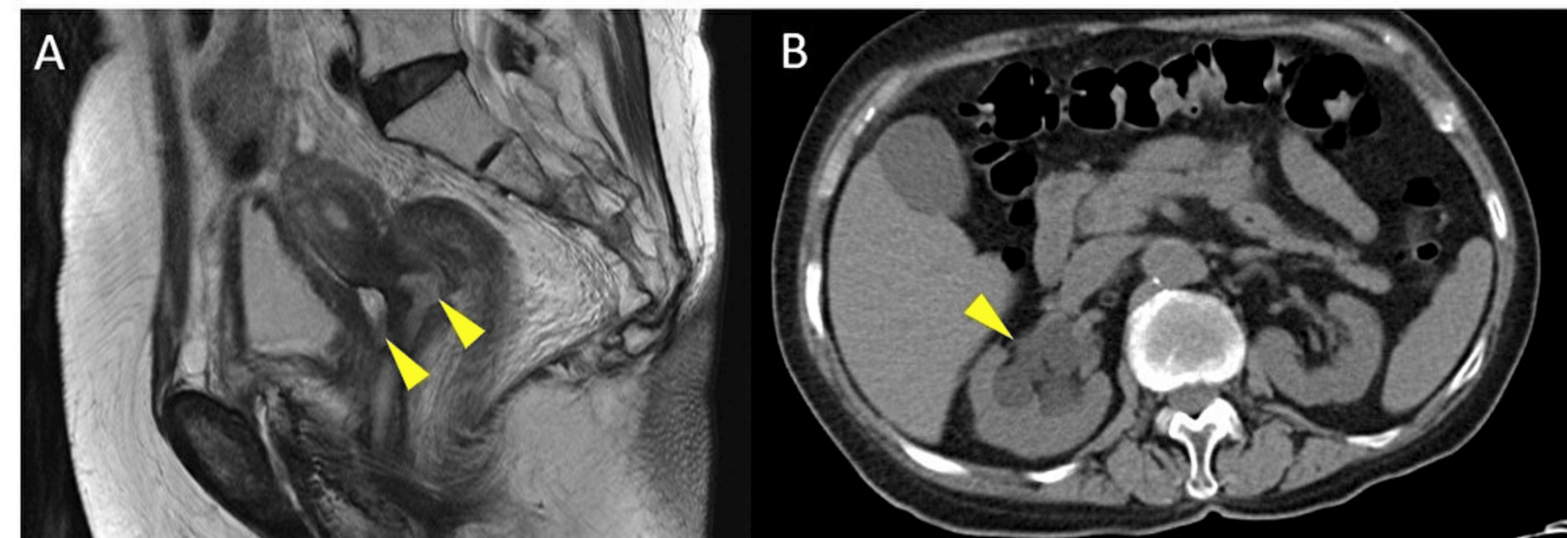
Magnetic resonance imaging and computed tomography A: MRI shows recurrent tumors in the uterine cervix and the vesicouterine pouch (arrowheads); B: CT shows hydronephrosis in the right kidney (arrowhead)
